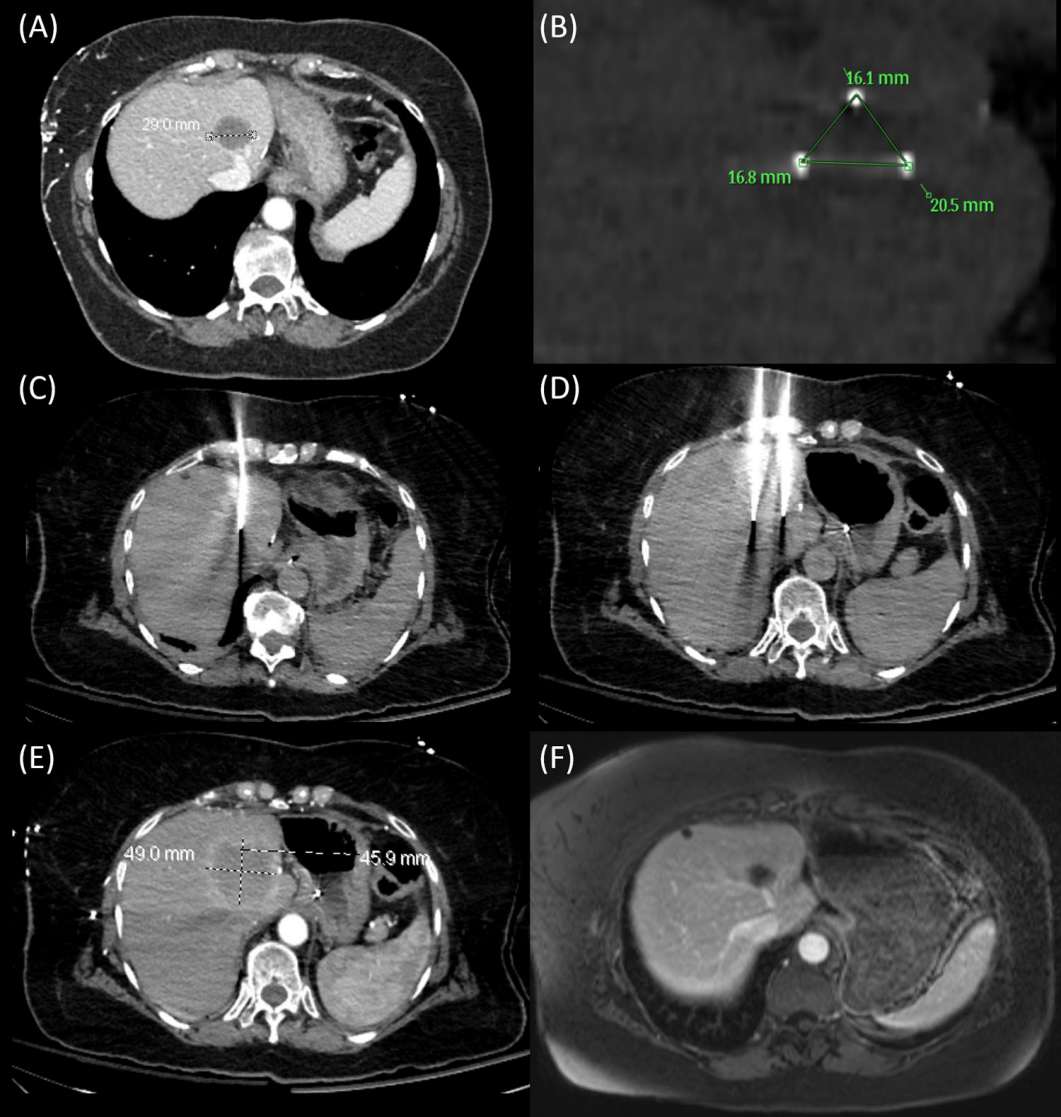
A Pre-procedural contrast-enhanced CT of the HEHE tumor in segment 4A measuring up to 29 mm. B Intraprocedural needle placement planning. C Intraprocedural CT showing intermittent placement of one out of three 17 gauge NanoKnife IRE ablation needles. D Intraprocedural CT showing intermittent placement of two out of three 17 gauge NanoKnife IRE ablation needles. E Intermediate post-procedural contrast-enhanced CT with an ablation zone measuring 46 mm × 49 mm. F Follow-up contrast-enhanced MRI after 8 months shows shrinkage of the ablation zone without residual enhancement
